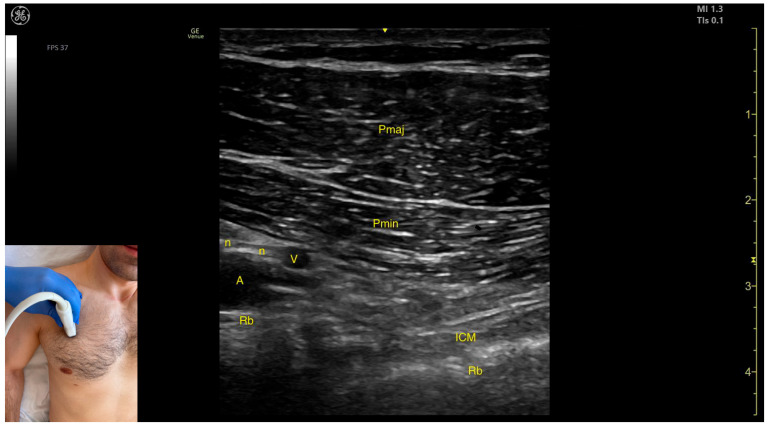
Ultrasound anatomy of the pectoralis minor (Pmin) with key landmarks: Pmaj—pectoralis major; Pmin—pectoralis minor; ICM—intercostal muscle; Rb—rib; n—nerve; A—artery; and V—vein.
